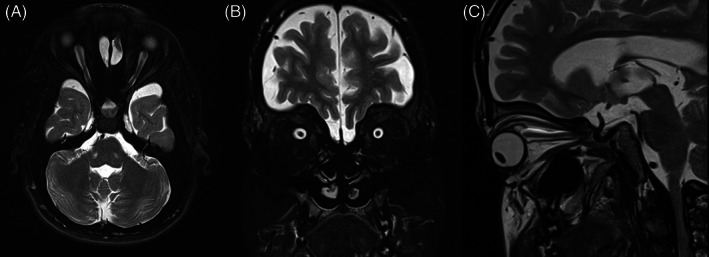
T2‐fat saturation MRI for patient 1 showing enlarged perioptic subarachnoid space. (A) Axial section; (B) coronal section; (C) sagittal section
